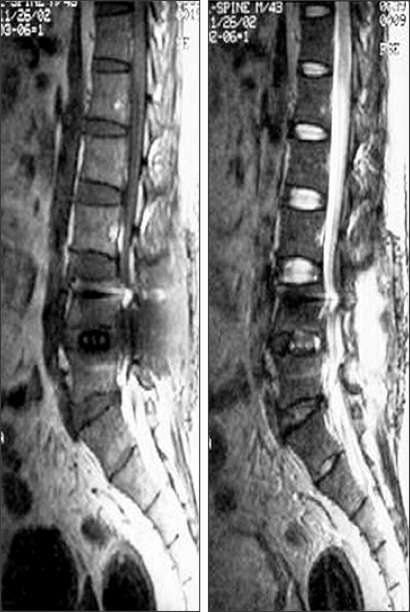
Sagittal T1- and T2-weighted images at 4 weeks post-operative. No definite sign of infection was identified.
